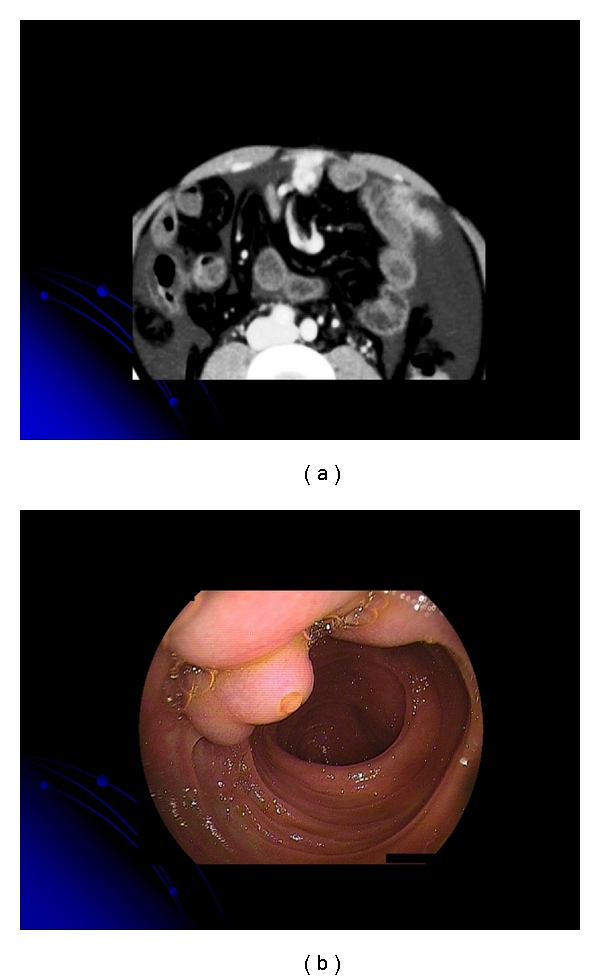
(a) Computed tomography showing the vessel image in the jejunum. (b) Double-balloon enteroscopy revealed jejunal varices with a white plug.
